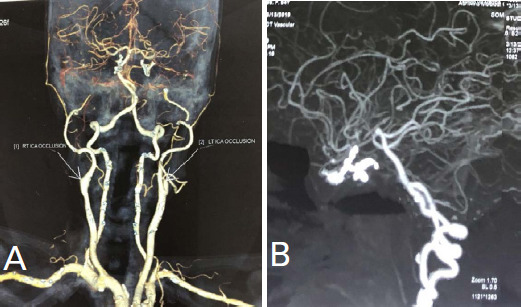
A) Computed tomography angiogram (CTA) demonstrating complete occlusion of bilateral internal carotid arteries just distal to common carotid arteries, B) Computed tomography angiogram (CTA) showing predominant cerebral vascular supply from posterior circulation.
